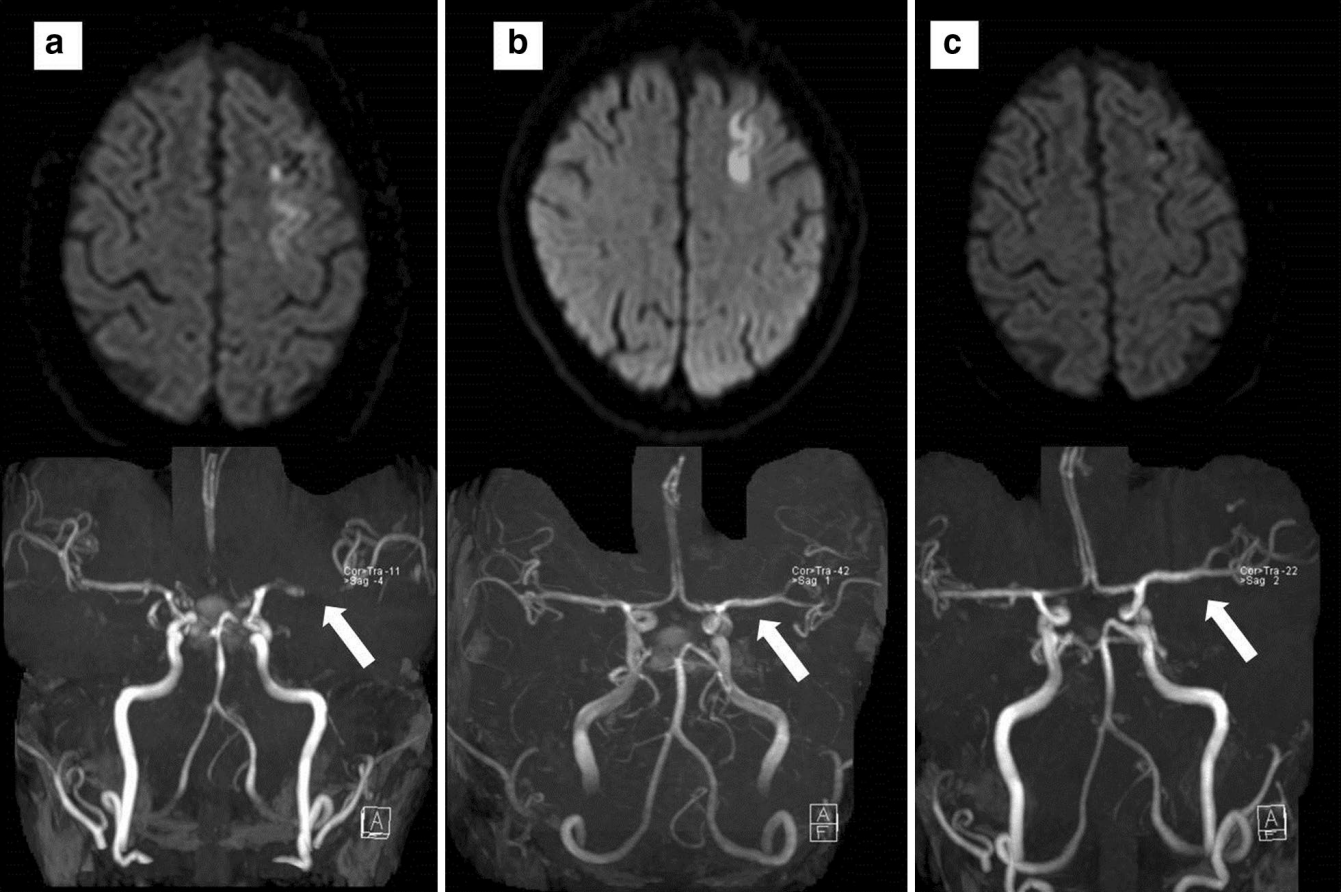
Axial magnetic resonance imaging demonstrating severe *left middle cerebral* artery vasospasm (time of flight angiography, *white arrow*) and *left frontal cortical* hyperintensities (diffusion-weighted imaging) before (a), 3 days after (b) continuous intra-arterial nimodipine treatment and at 23d follow up (c)
